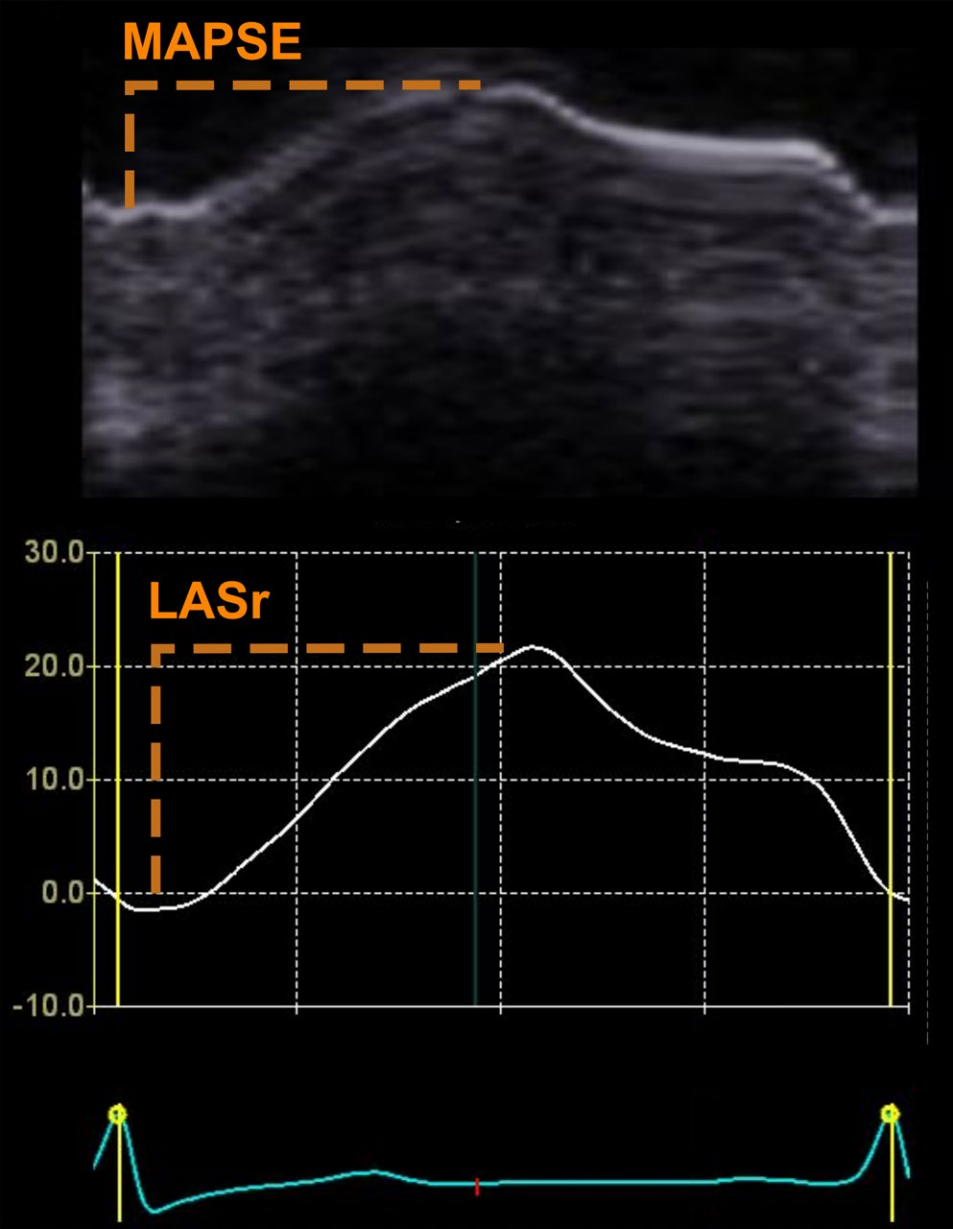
Mitral annular plane systolic excursion (MAPSE, upper panel, orange) and left atrial reservoir strain (LASr, lower panel, orange) measurements from echocardiography. Notice the close relation between MAPSE curves and LASr. The amplitude of the LASr depends on the left atrial size
